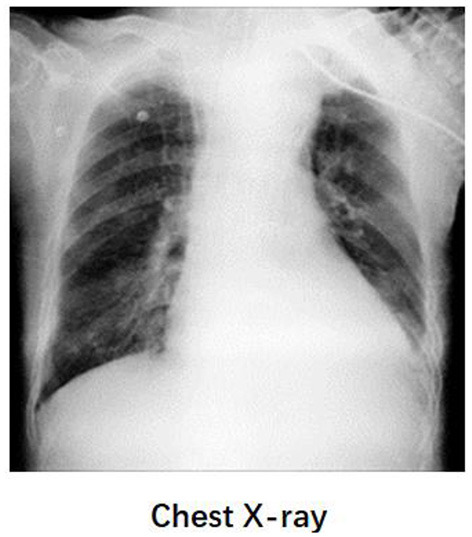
The chest X-ray of patient. Chest radiography did not show signs of pneumothorax. Functional EIT images of ventilation showed that the defect of the left lung had been restored after treatment.
